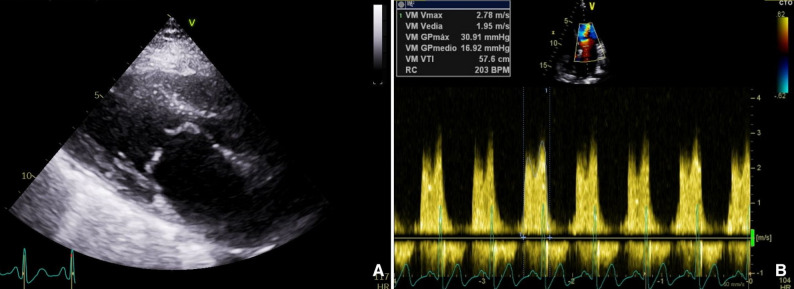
Transthoracic echocardiogram. A Parasternal long-axis view. Mitral valve with diffuse thickening of both leaflets, restriction in their marginal opening with bicommissural fusion, minimal calcification. B Spectral Doppler. Mitral stenosis with a maximum velocity of 2.8 m/s, a peak gradient of 31 mmHg and mean gradient of 17 mmHg. Mild mitral regurgitation
